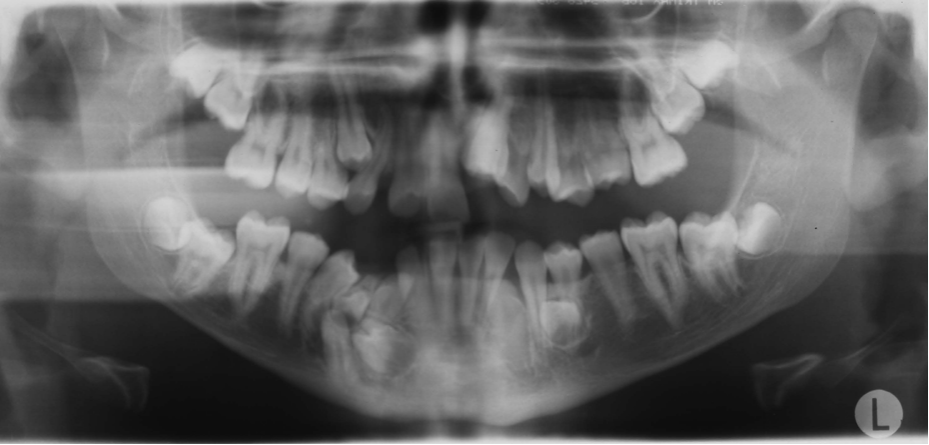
Orthopantomography at 14 years 1 months of age.
